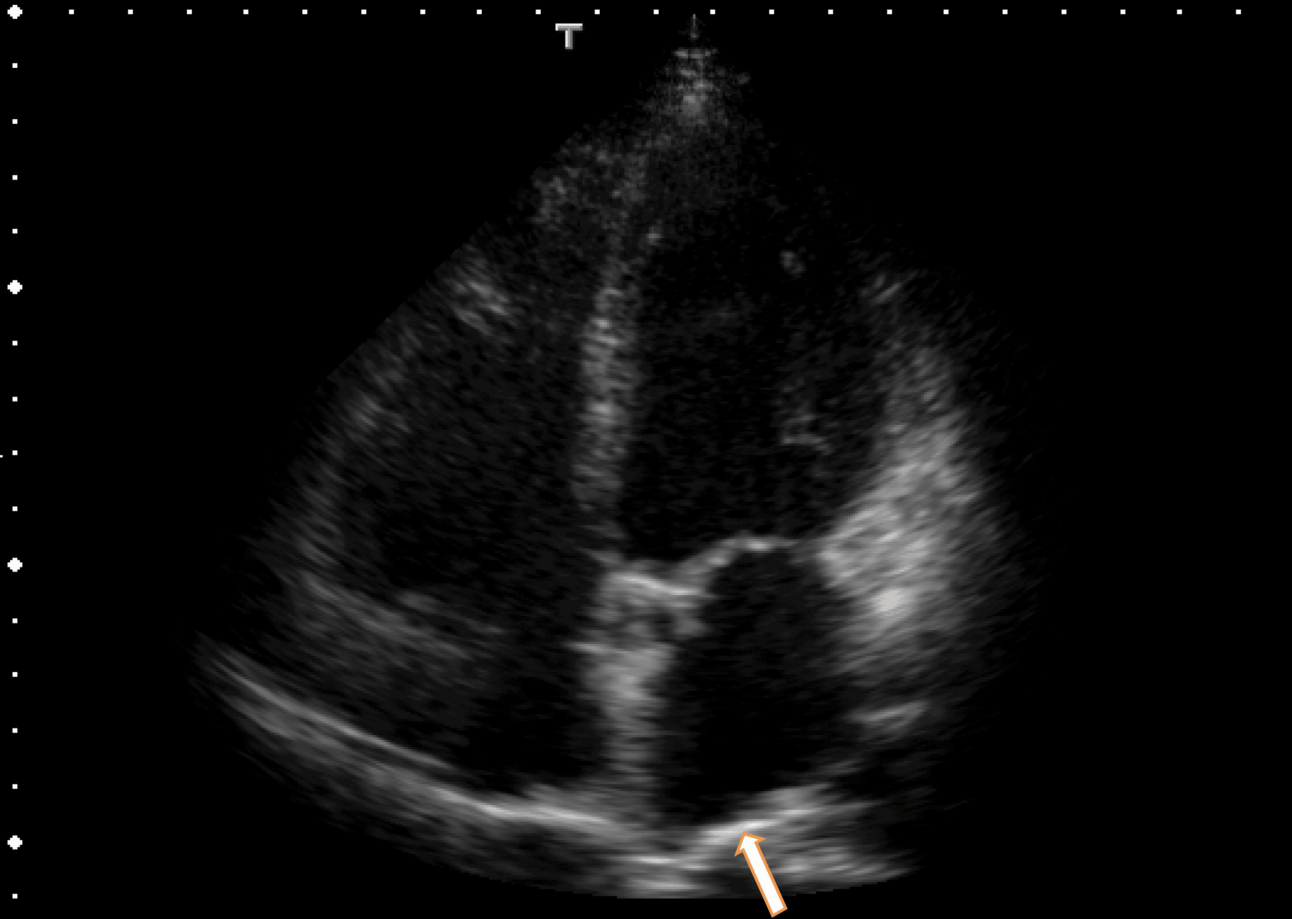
Transthoracic echocardiography (TTE) images showing left atrium (LA) thrombi TTE images showing a hyperechoic region in the LA around the base of the right upper pulmonary vein (RUPV) (arrow).
